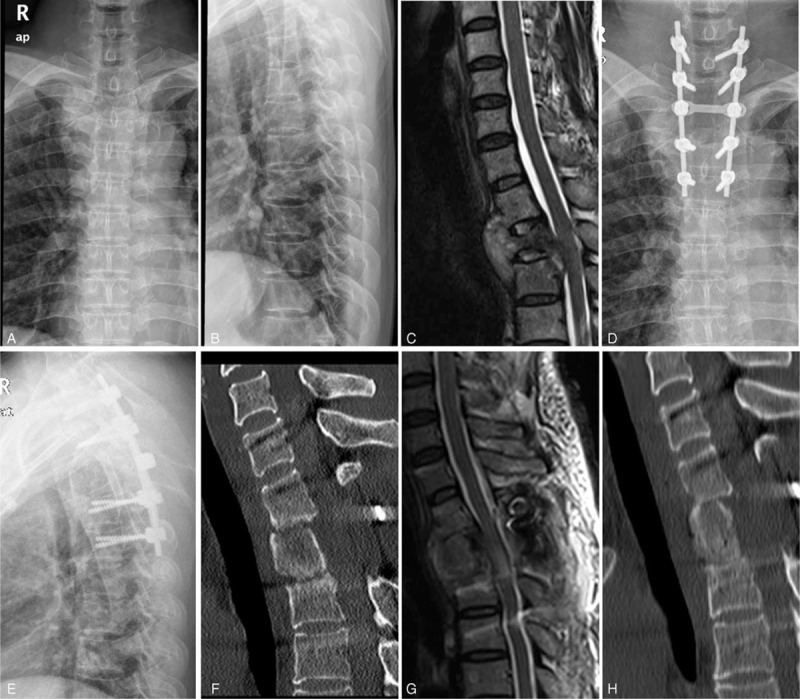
Preoperative anteroposterior and lateral X-ray of a 39-year-old female with tuberculosis of T2, who presented with a local kyphosis angle of 20° (A, B). Sagittal T2 WI (C) images showed active tuberculosis with abscess formation, vertebral destruction, and cord compression. This patient was treated by the posterolateral approach with pedicular screw-rod fixation. Postoperative X-rays (D, E) of the same patient showed good decompression and kyphosis correction, with a kyphosis angle of 9°. Postoperative sagittal thoracic CT imaging showed good positioning of the grafting iliac bone (F). Postoperative sagittal T2 WI (G) images showed no significant residual lesions around T2 and complete spinal cord decompression. At the 8-month follow-up examination, a sagittal thoracic CT revealed solid bony fusion, no loss of kyphosis correction, and no obvious recurring lesions around T2 (H).
